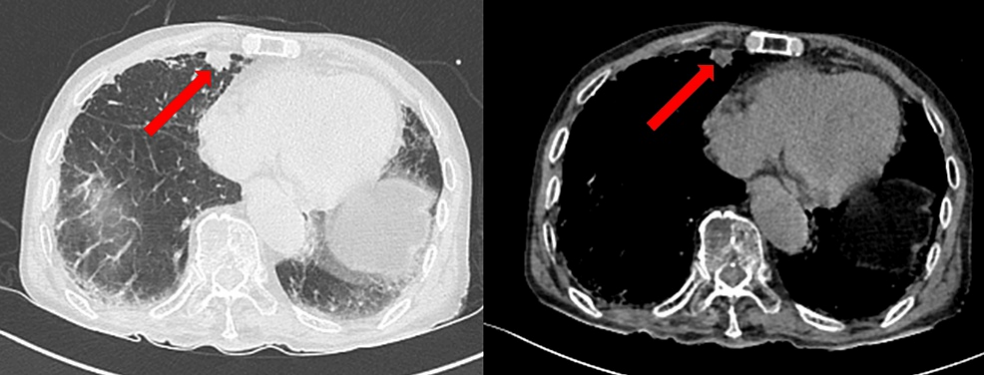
Chest CT showed a mass image on the mediastinal side of the lower middle lobe (red arrow) and no obvious pneumonia.
